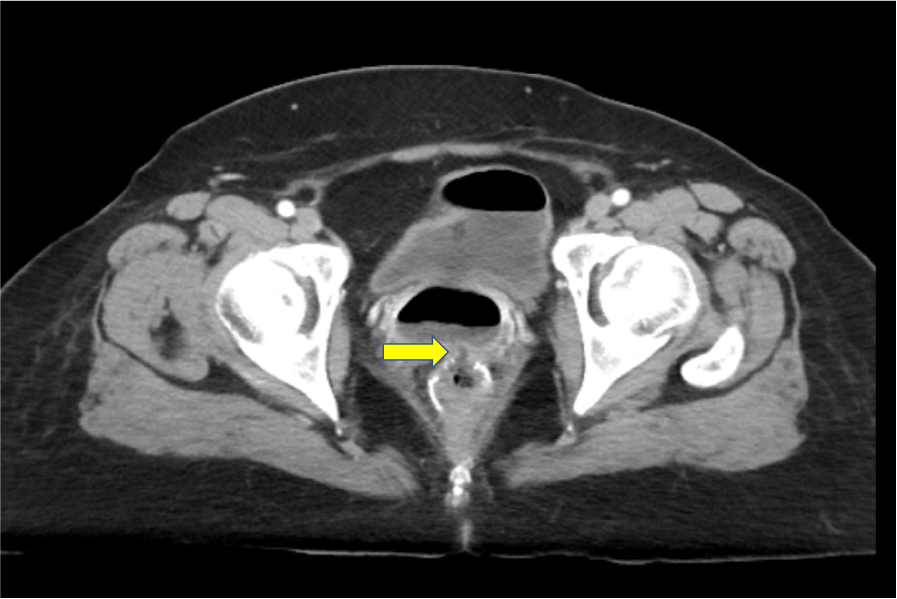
Computed tomography scan revealed a fistula between the vagina and the rectum (arrows)
